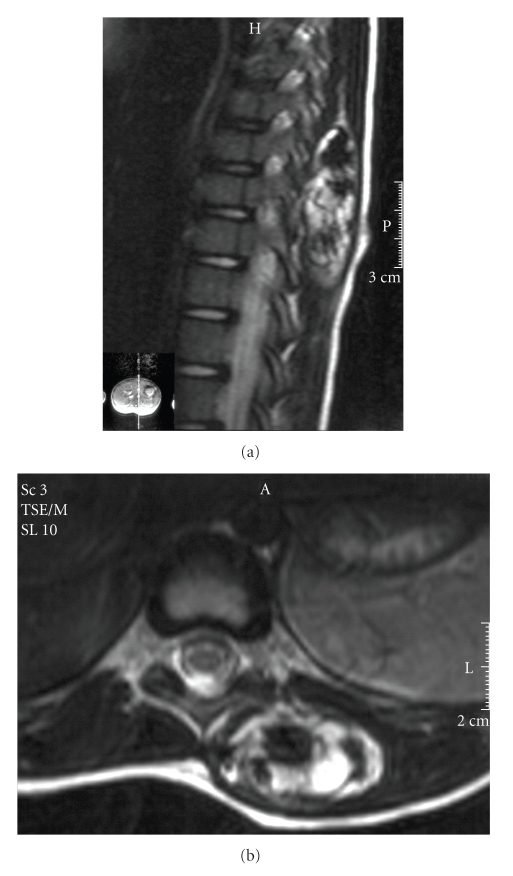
Sagittal and axial T2 weighted MRI images demonstrating a well-defined paraspinal mass.
